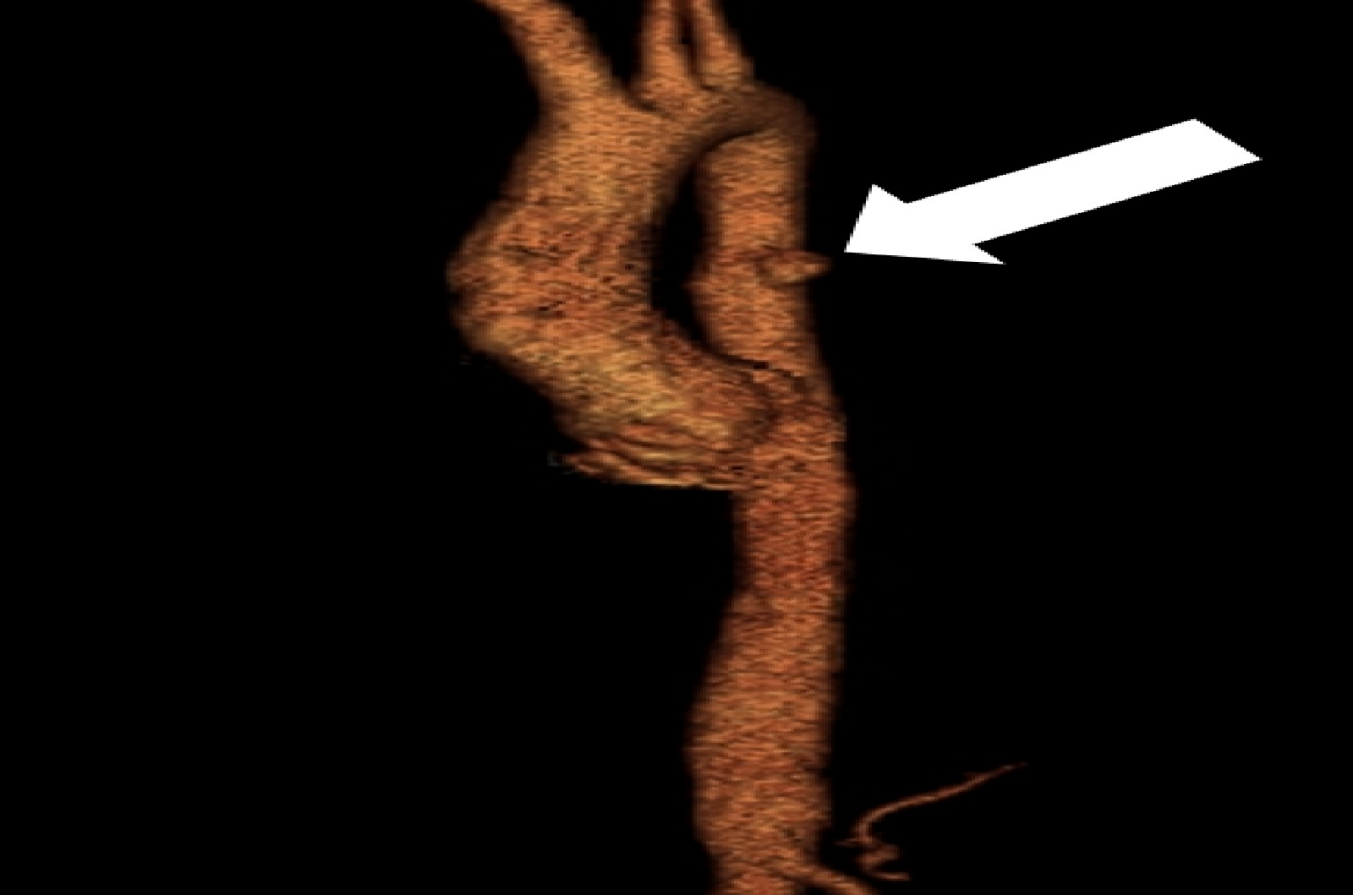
Volume-rendered computed tomography angiogram (CTA) demonstrating the penetrating ulcer (arrow).
